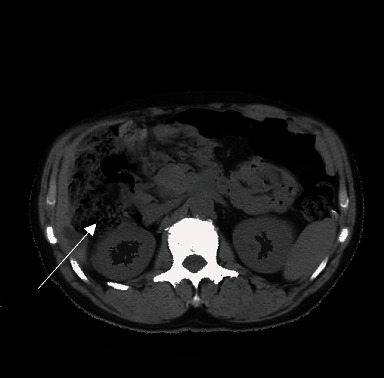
CT image of lymphomas. The white arrow suggested the lesion with even density and continuous mucosal line.
